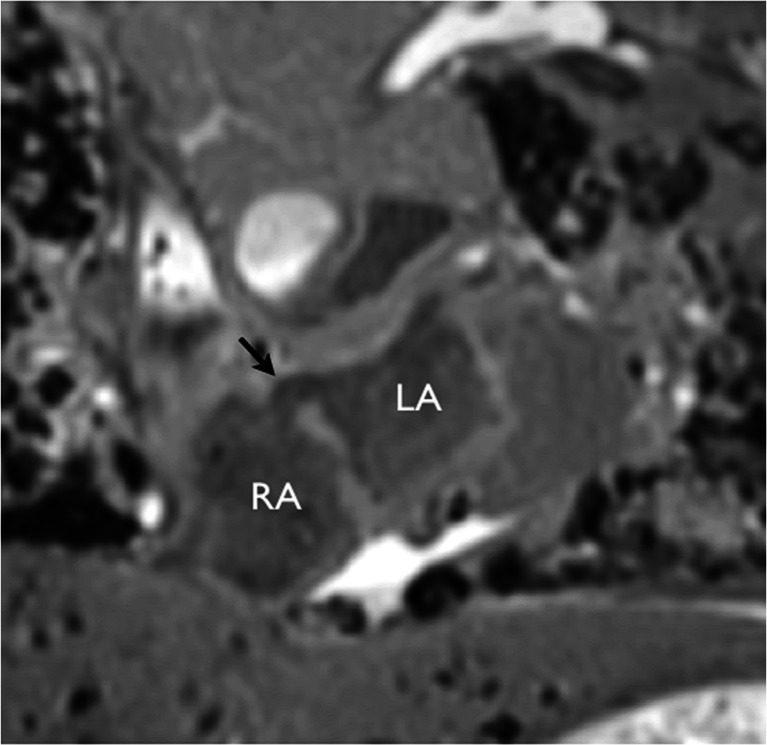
Potential overcall by cardiac postmortem MRI. A high atrial septal defect (*arrow*) was called on coronal T2-weighted postmortem MRI. Conventional autopsy of the heart was reported as normal. *LA* left atrium, *RA* right atrium
